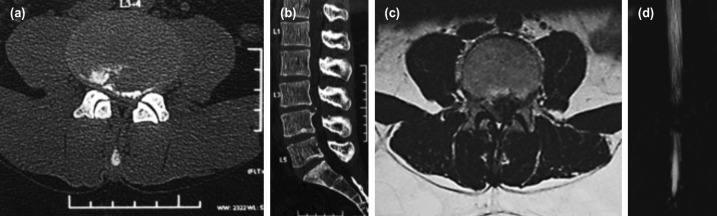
(a) Pre-operative CT (Computed Tomography) scan axial (b) and sagittal, (c) axial MRI in a 23-year-old male patient of CES with bilateral leg affection and vesicular dysfunction showing a calcified central LDH (Lumbar Disc Herniation). (d) MRI myelogram showing complete block.
